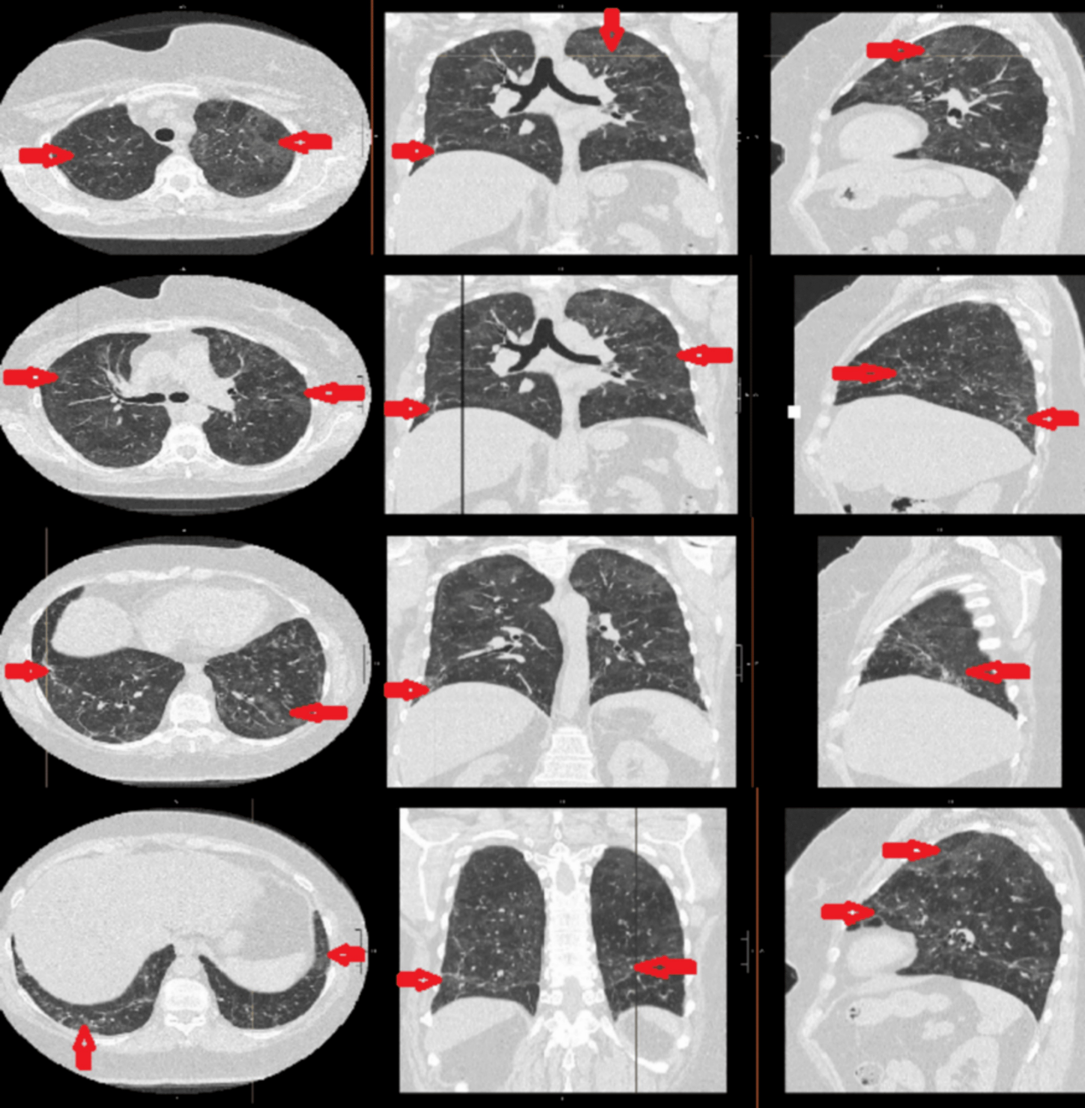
Axial, coronal, and sagittal views of CT of the chest without contrast at four different levels demonstrate diffuse, symmetric lung disease with ground-glass and irregular linear opacities, highlighted by red arrows
